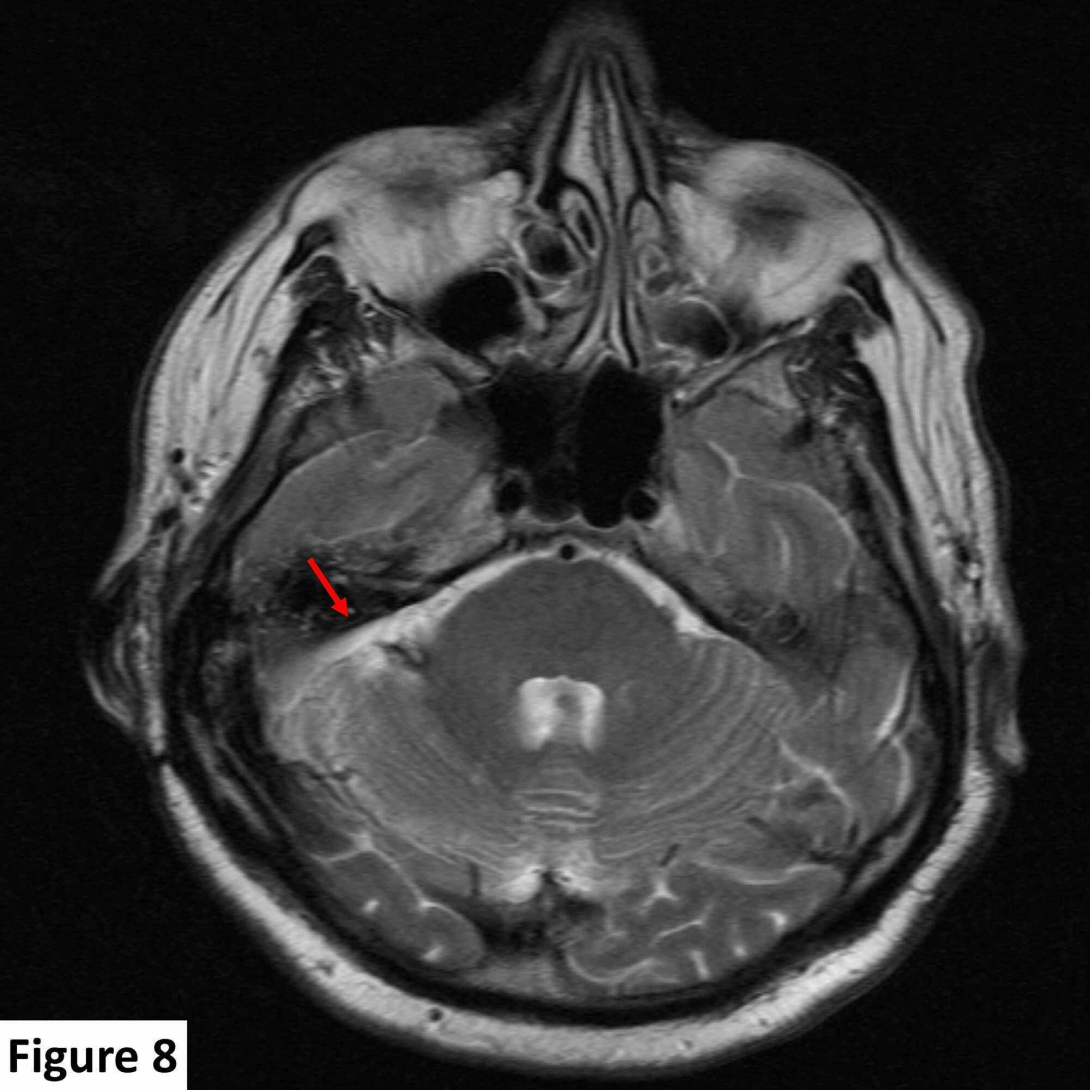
Follow-up axial T2 sequence MRI image. At 12 months postoperative follow-up there was no evidence of tumor recurrence (red arrow). MRI, magnetic resonance imaging
